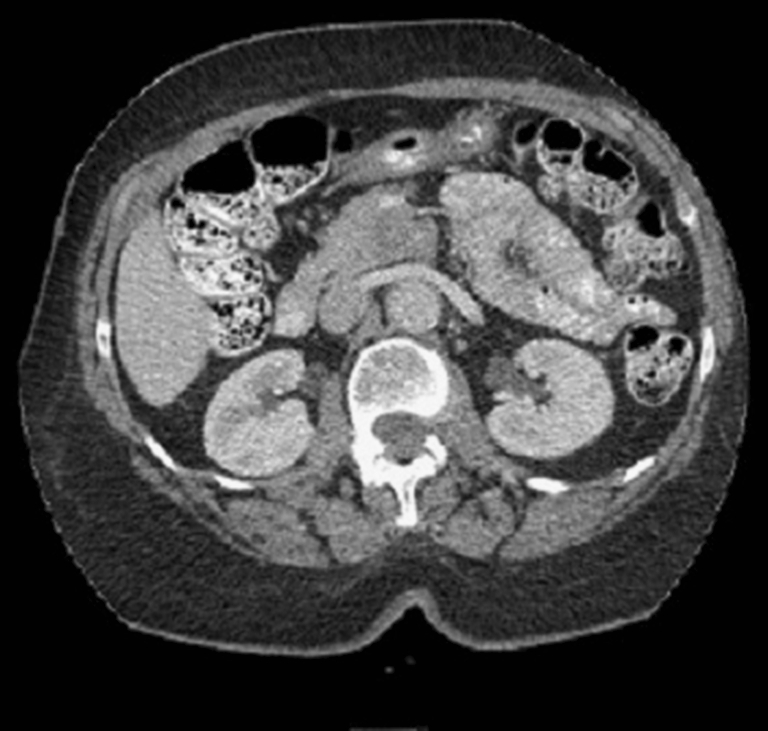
Computed tomography demonstrating a subtle mass in the head of the pancreas, posterior to the portal confluence
